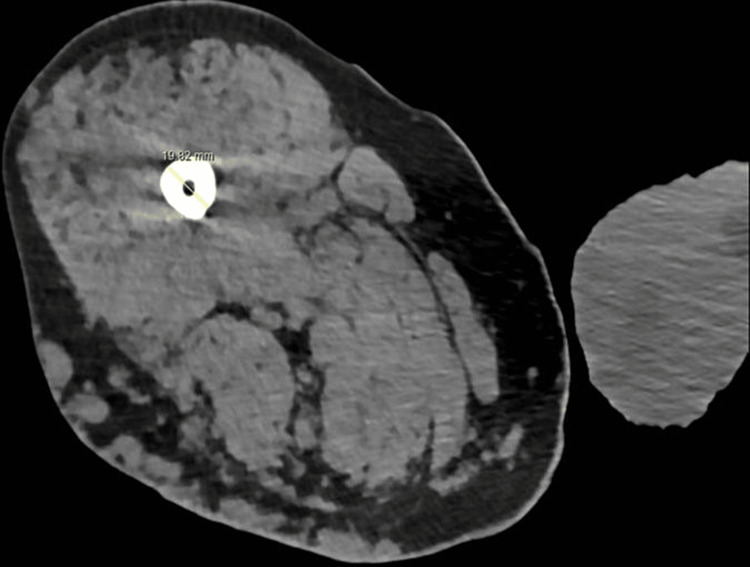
CT scan showing narrow femoral canal
